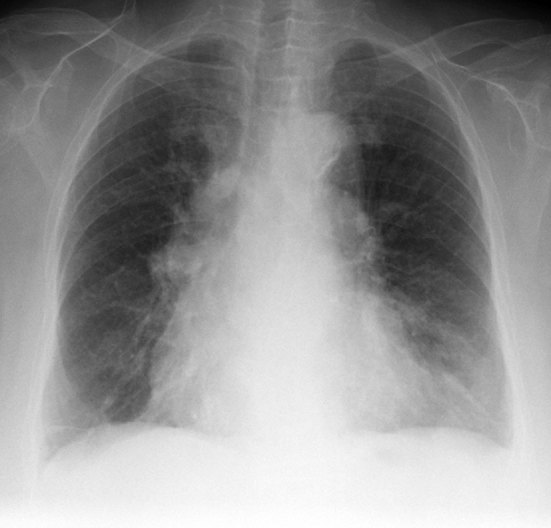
Chest radiograph shows a vague elongated opacity in the left lower lung.
